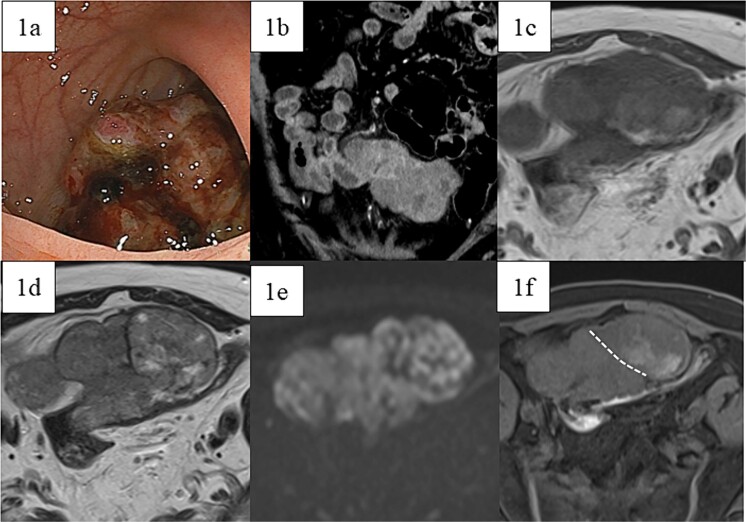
Preoperative images. (a) Lower gastrointestinal endoscopy revealed a type 1 tumor in the sigmoid colon. (b) Contrast-enhanced computed tomography showed a contrast-enhanced soft-tissue tumor measuring 80 mm × 95 mm × 45 mm (arrow), which was partially adherent to the small intestine. MRI showed a tumor that was heterogeneous in both (c) T1- and (d) T2-weighted images with predominantly low-intensity areas, with some high-intensity areas in T2. (e) Diffusion-weighted images showed high intensity throughout the entire tumor. Contrast-enhanced MRI (f) showed two areas with slightly different contrast effects. White dotted lines indicate boundaries of the areas with different contrast effects.
